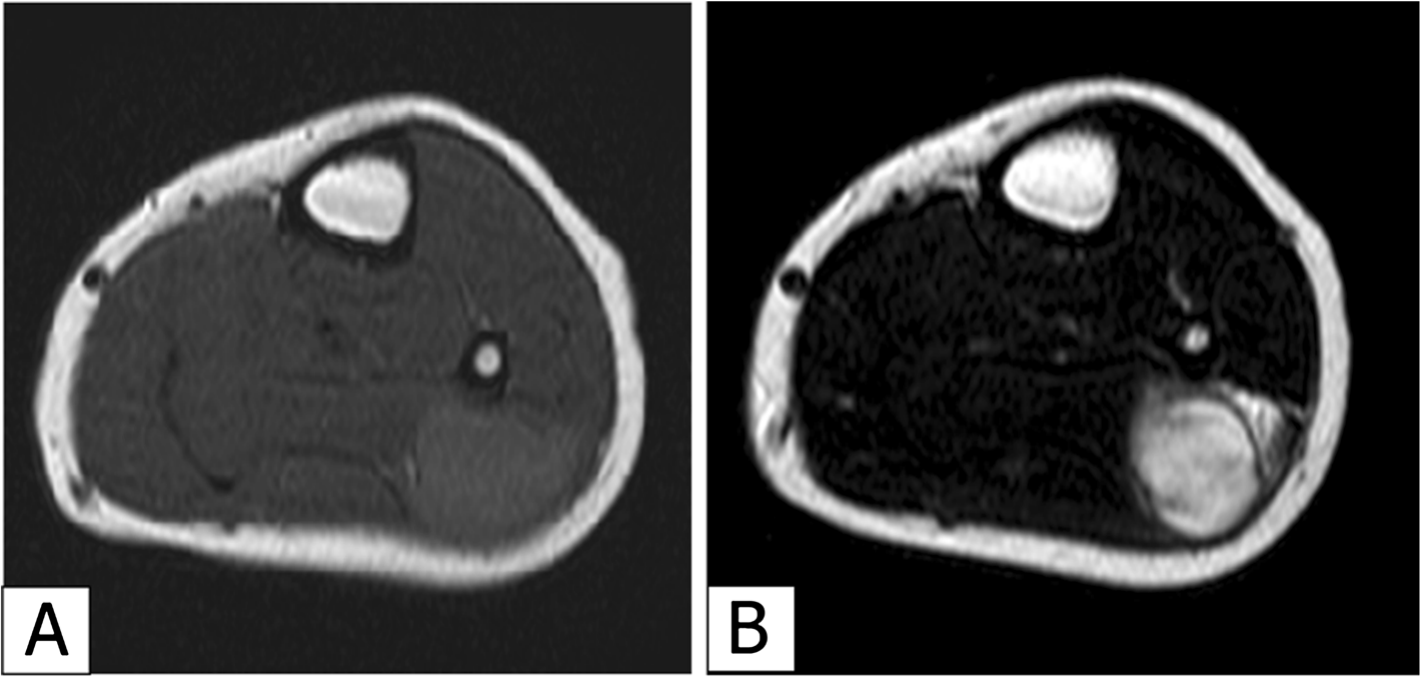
MRI of lower left leg of the 5-year-old patient. An axial view MRI revealed a well-defined mass measuring 27 × 20 mm in the lateral aspect of the gastrocnemius. The mass had slightly high intensity compared to the skeletal muscle on T1WI (A) and a heterogeneously high intensity on T2WI (B).
